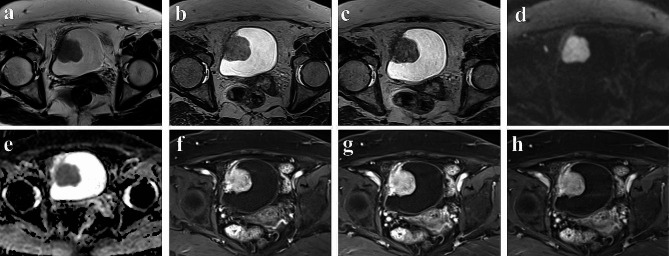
(a-h) display a bladder tumor located in the right wall of the bladder, pathologically diagnosed as muscle invasion. Axial T2WI (a), T2-weighted-SPACE (b), CS-T2-weighted-SPACE (c), DWI (d), ADC (e), arterial phase (f), intravenous phase (g) and delayed phase (h)
